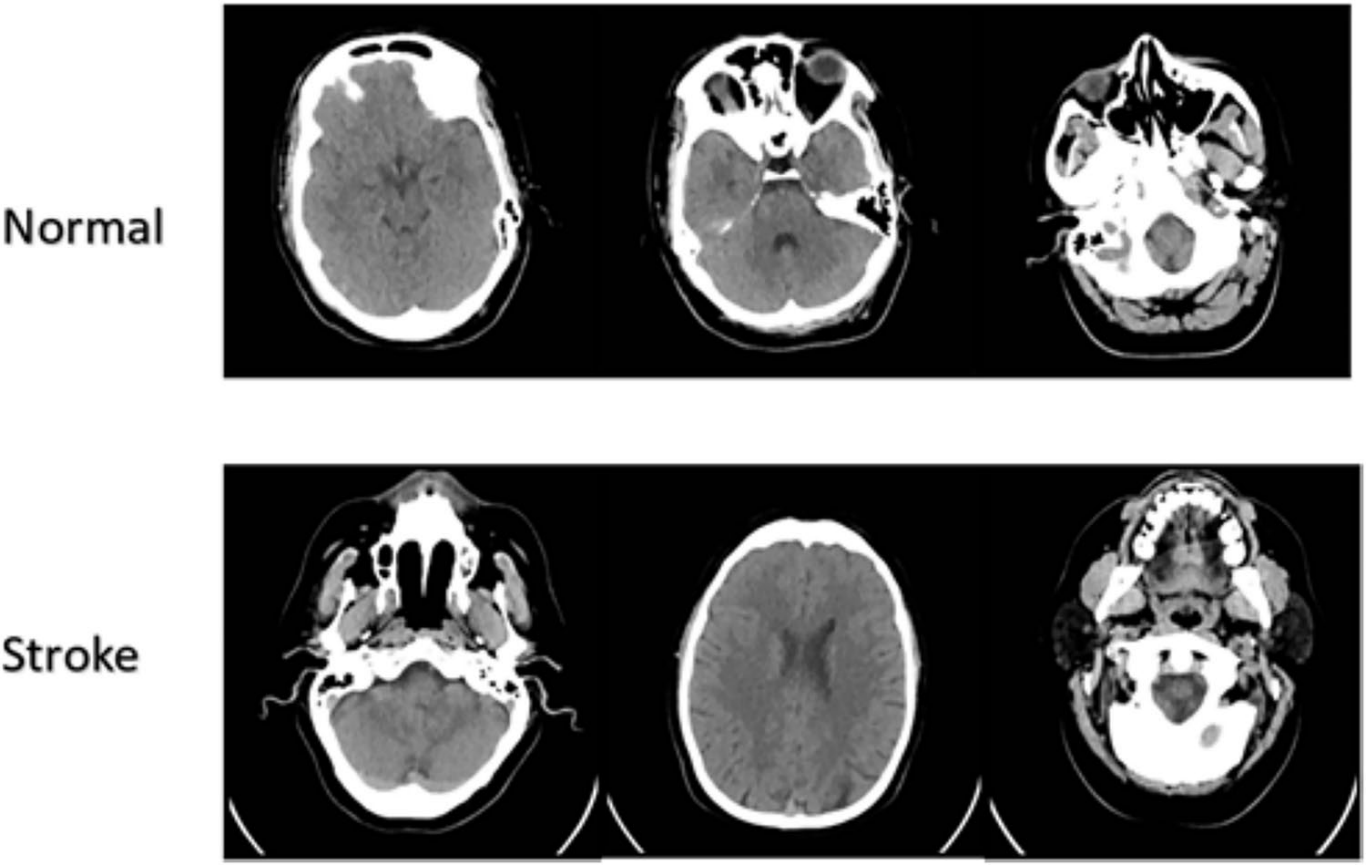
Sample images showing normal and stroke-affected brain CT scans used for model training and evaluation.
